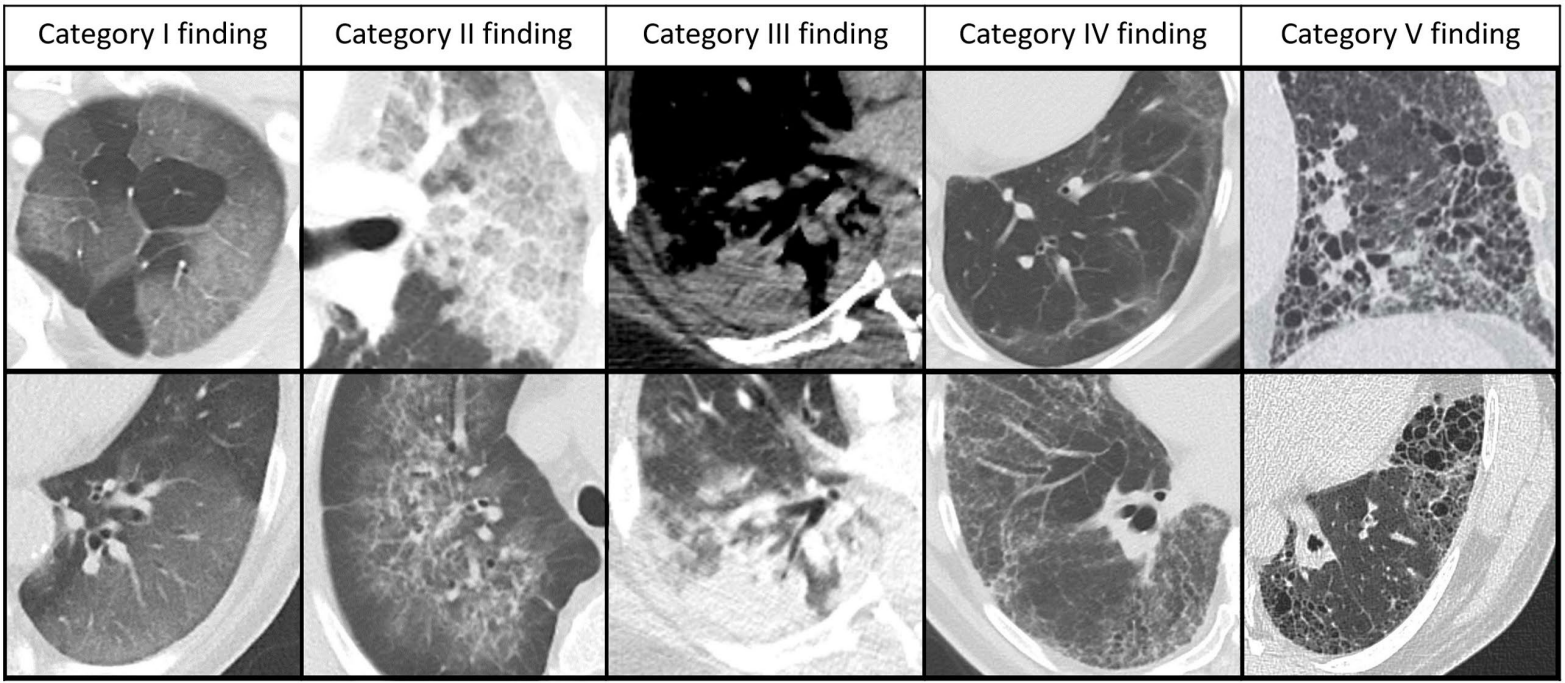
Chest CT findings categories of the RACOON Viral Pneumonia Score. This figure shows five different CT findings categories, which are in principle non-specific but have been described consecutively in the staged course of COVID-19 pneumonia and are therefore subscores of the RACOON Viral Pneumonia Score (RVPS). From left to right: Category I findings comprise pure ground glass opacities (GGO). Category II findings comprise GGO superimposed by interstitial septal thickening, which may result in a crazy paving pattern. Category III findings comprise consolidations, which are often surrounded by GGO. Category IV findings comprise diverse, potentially reversible, post-pneumonic findings such as septal lines, parenchymal bands, and reticulations, all of them without traction or architectural distortion. Category V findings comprise fibrotic-like or cystic changes that are considered irreversible sequelae of pneumonia: Reticulations with traction of the surrounding lung parenchyma and bronchi as well as cystic lesions such as honeycombs and pneumatoceles. Bronchus dilatations can be observed in any phase of pneumonia and are therefore not included in the RVPS.
